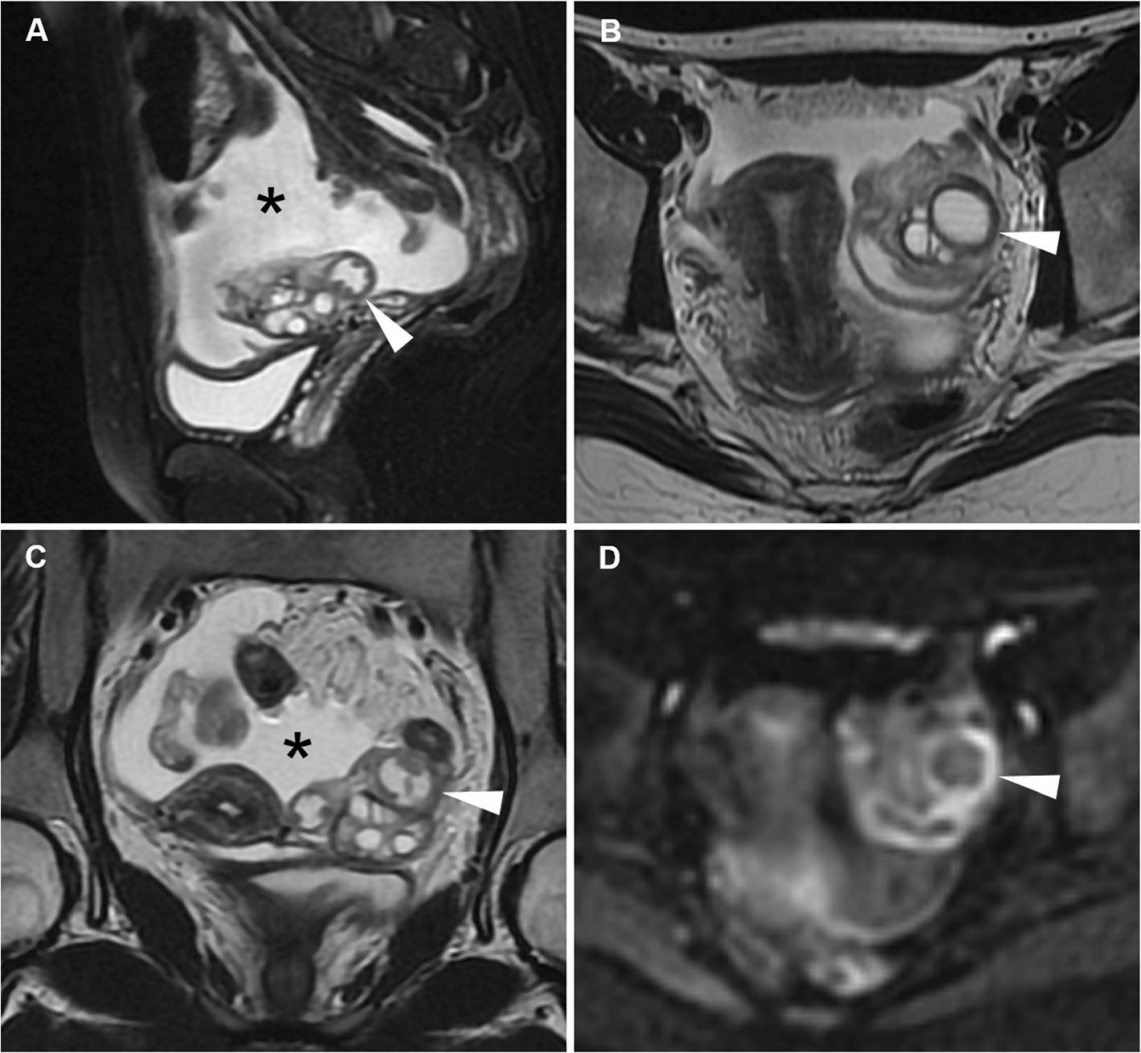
Acute salpingitis in a 25-year-old woman with pelvic pain and mild neutrophilic leukocytosis. Sagittal fat-suppressed (a), oblique-coronal (b) and oblique-axial (c) T2-weighted images show slightly dilated left fallopian tube with thickened walls (arrowheads). On axial diffusion-weighted imaging (DWI, *b* = 800 s/mm^2^) image (d), the tubal wall thickening demonstrates restricted diffusion (arrowheads), finding consistent with acute inflammation. Note pelvic peritoneal effusion (asterisk)
